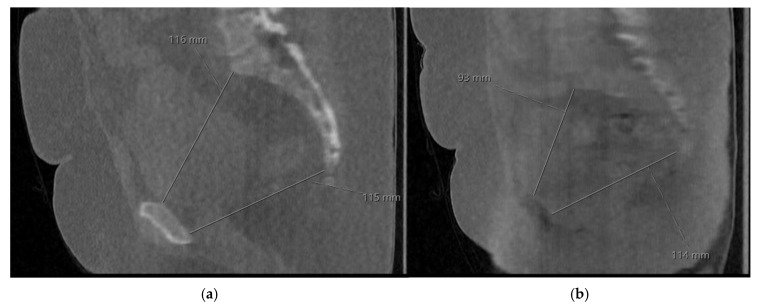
Examples of CT pelvimetry cases with (a) clearly sufficient image quality and (b) low image quality, making it difficult to define the sacrum and coccyx (tailbone). The measures shown are the medial obstetric conjugate (inlet) and the sagittal pelvic outlet diameter.
